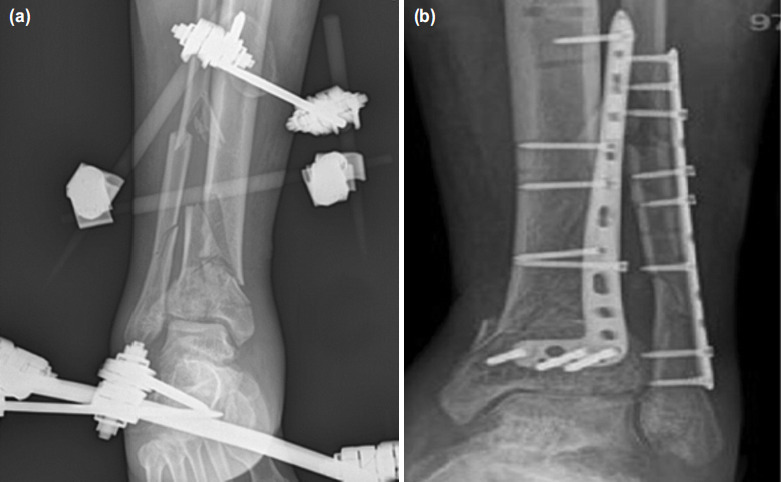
(a) Radiograph images of the patient who did not have fibula fixation in the first stage. In the first stage, only external fixator was applied for fractures of the tibia and fibula. (b) Definitive treatment was applied to the fracture of the tibia and fibula after soft tissue swelling resolves.
